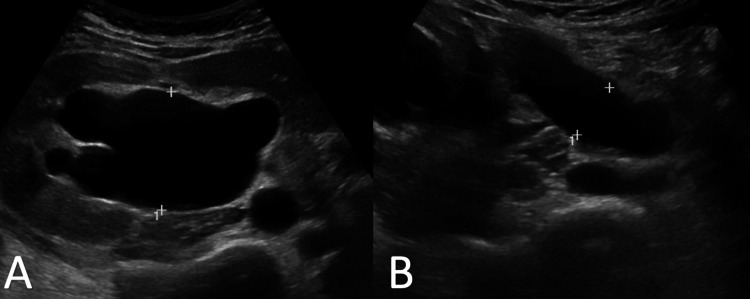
Initial abdominal ultrasound. A. Ultrasound image showing anteroposterior dilatation of renal pelvis of 4.4 cm. B. Ultrasound image showing ureteral dilatation of 1.87 cm.
